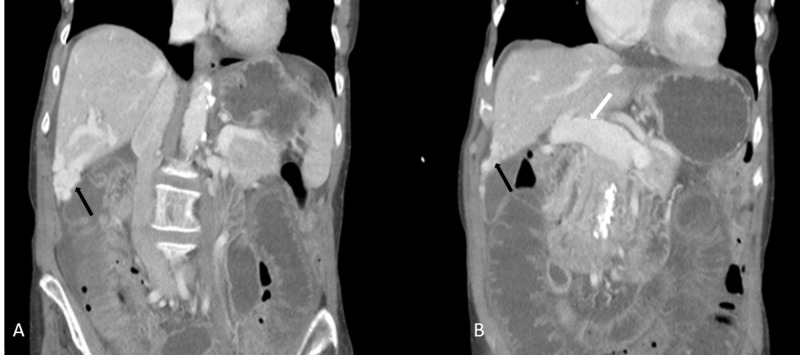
Coronal images of contrast-enhanced CT of the abdomen (A) A coronal view showing multiple dilated vascular channels (black arrow) in the right hepatic lobe (segment V). (B) Another coronal view which also shows dilated portal vein measuring up to 1.8 cm at the porta hepatis (white arrow) and multiple dilated vascular channels (black arrow) in the right hepatic lobe (segment V).
